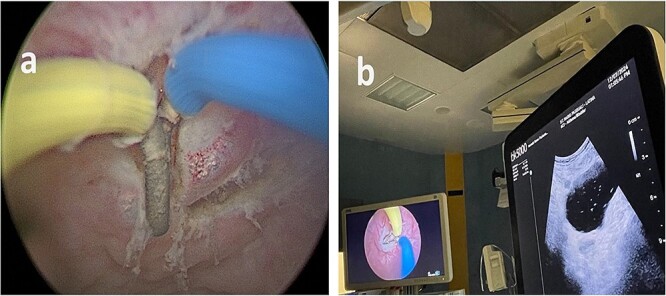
(a) Endoscopic vision during resection. (b) Endoscopic and ultrasound contemporary vision of the lesion.
